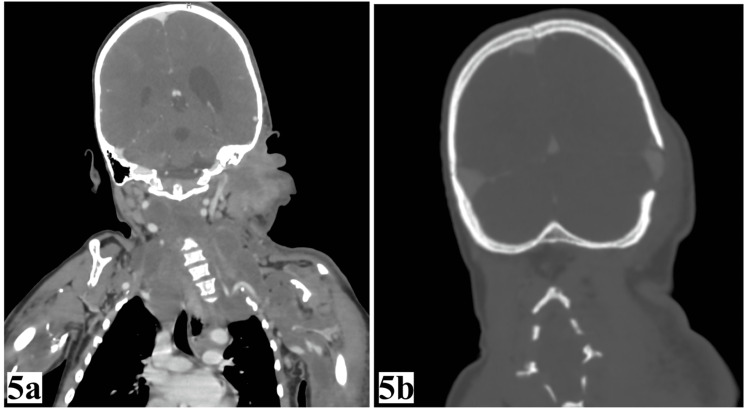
CT scan of the patient. (a) Cervicothoracic kyphoscoliosis; (b) diastasis of left lambdoid suture.
